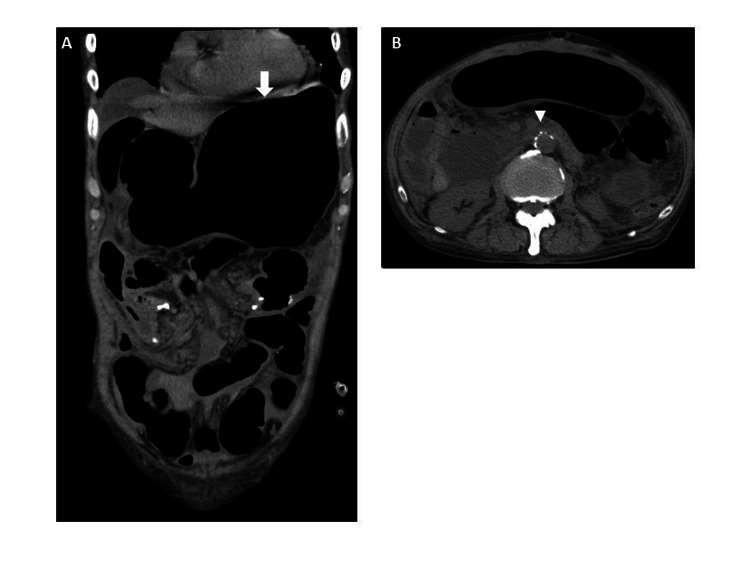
Findings of the CT scan performed on the seventh postoperative day (A) Coronal CT findings showed that the stomach (white arrow) was highly dilated, but no obstruction was observed in the pelvic small intestine and colon. (B) Axial CT findings showed that the aorta-mesenteric distance (white arrowhead) was 4.4 mm. CT, computed tomography
